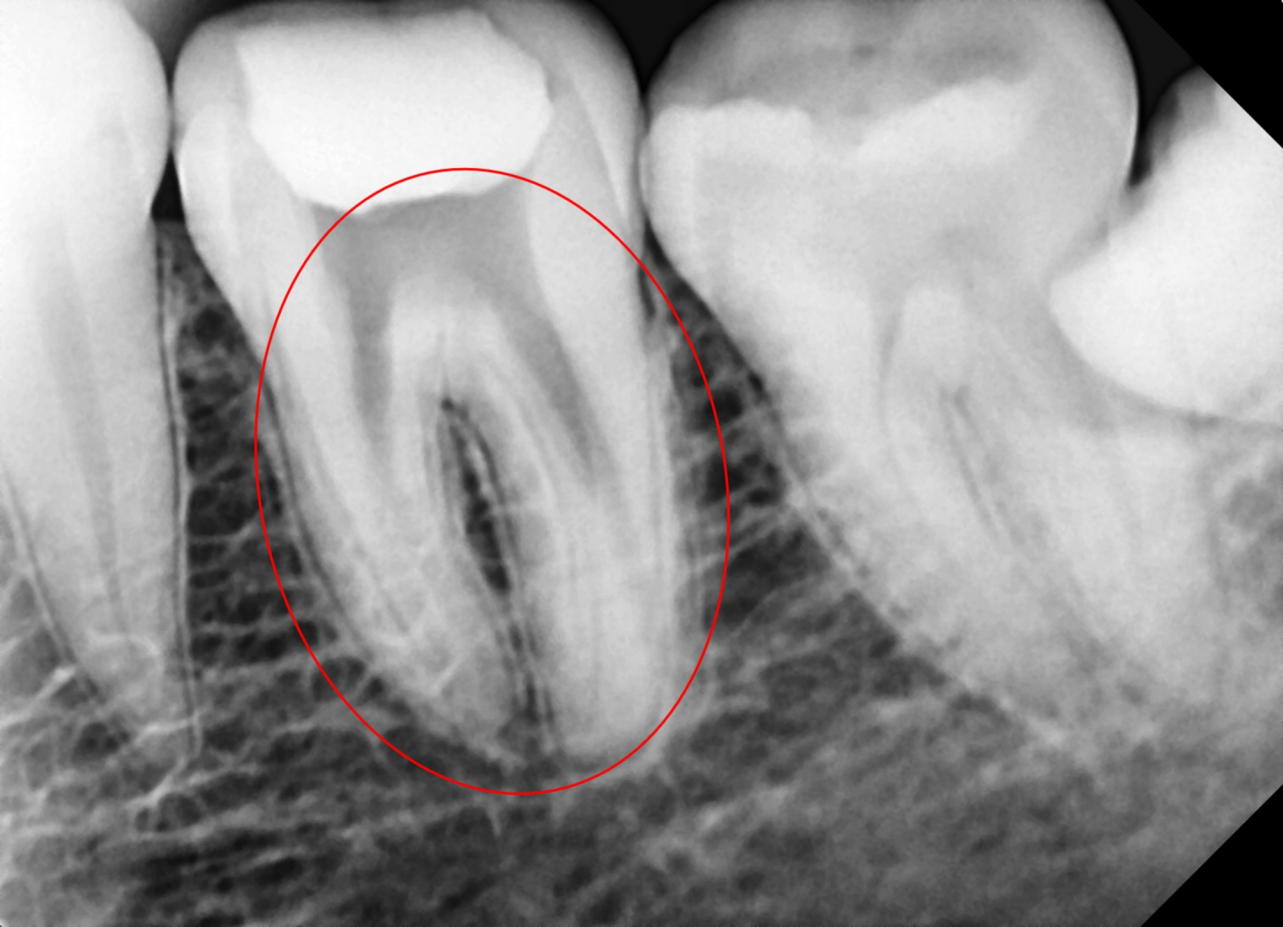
Baseline digital radiograph
